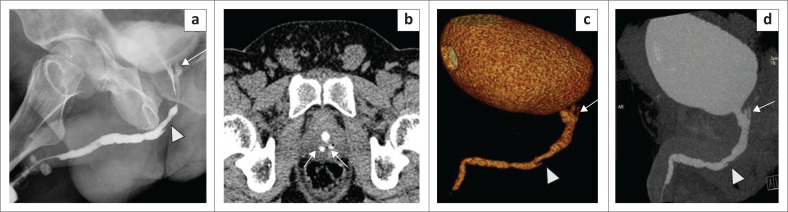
(a) Retrograde urethrography (RGU) image (right lateral view) showed an outpouching from the prostatic urethra (arrow), likely a urethral diverticulum. Anterior urethritis and a false tract (arrowhead) in the bulbar urethra were also noted. (b) Axial section (multidetector computed tomographic urethrography [MDCTU]) of the same patient showed reflux into both ejaculatory ducts (arrows), which mimicked the appearance of urethral diverticulum on RGU. (c, d) Volume rendered technique (VRT) and maximum intensity projection (MIP) images, respectively (MDCTU), of the same patient also showed reflux into bilateral ejaculatory ducts (arrows), which mimicked the appearance of a urethral diverticulum on RGU. Anterior urethritis and a false tract in the bulbar urethra (arrowhead) were also noted on VRT and MIP.
